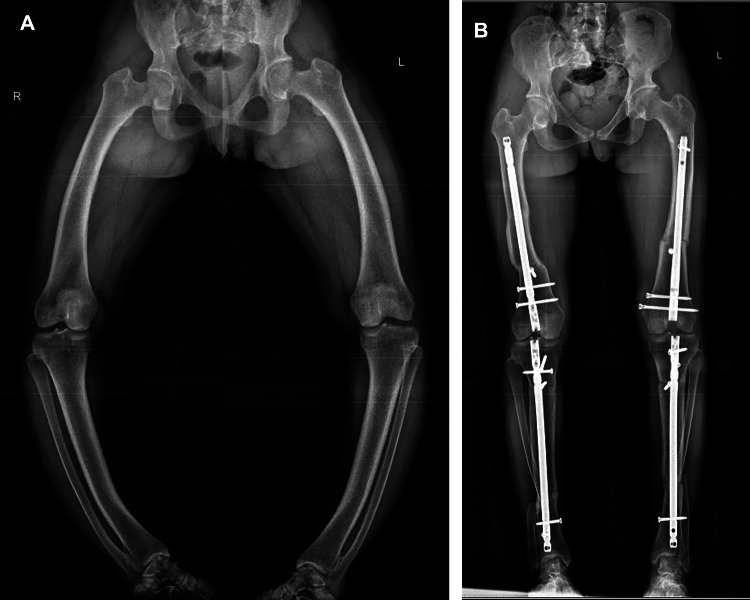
case of varus alignment where femur and tibia equally contribute to the deformity; A: pre-op standing full-length radiographs, B: full-lengthen standing radiographs at final fallow-up
